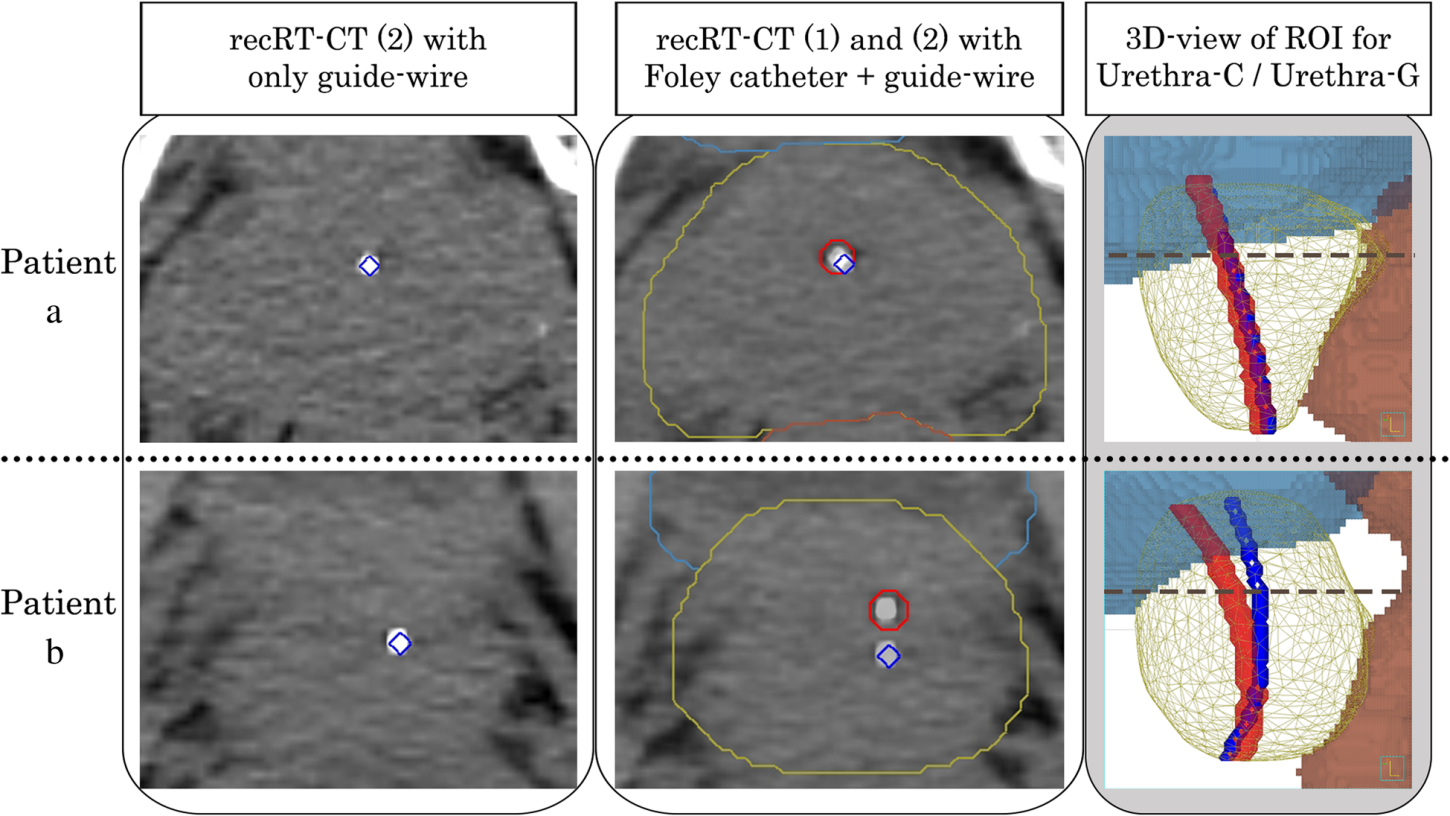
Representative patients with a small and with a large “C-G displacement”. The left image shows the recRT-CT (2) with urethra-G (blue). The middle image shows the fusion image of recRT-CT (1) and recRT-CT (2) with urethra-G (blue) and urethra-C (red). The right image shows the 3D view of urethra-G and urethra-C with the ROI for the prostate (yellow), bladder (blue), and rectum (brown). The broken line (dark blue) shows the height level of the RT-CT slices in the left and middle images. The “C-G displacement” was small throughout the whole prostatic urethra in patient a. On the contrary, the “C-G’ displacement” was apparent at the middle and large at the superior segment in Patient b
